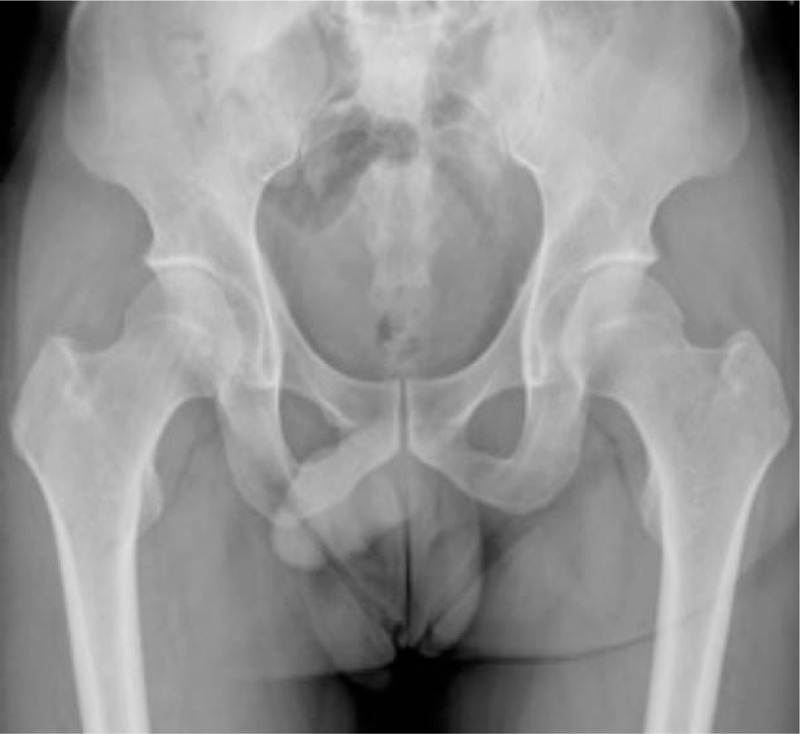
Anteroposterior radiograph of bilateral hips showing normal bony structure 48 days after corticosteroid therapy for idiopathic deafness.
